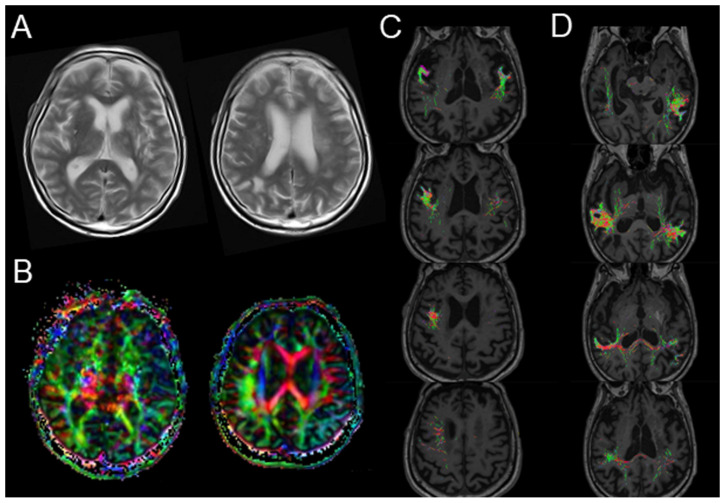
Postoperative MRI performed 2 weeks after reperfusion therapy. (A) T2-weighted brain MRI showed no new lesions. (B) Three-dimensional anisotropy contrast images showed darker areas in the left perisylvian cortex and lateral temporo-parietal lobe compared with those on the contralateral side. The fractional anisotropy map of the arcuate fasciculus using diffusion tensor imaging tractography-based anatomical region-of-interest seeding in Broca’s (C) or Wernicke’s area (D).
